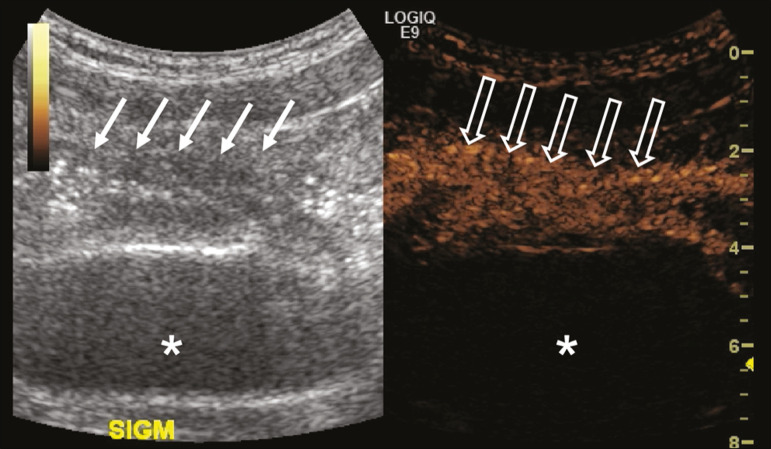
A 15-year-old patient, under follow-up treatment for Crohn’s disease, with abdominal pain and suspected exacerbation of the disease. Images after intravenous injection of microbubble contrast medium, showing wall thickening of the sigmoid loop (arrows) on B-mode ultrasound; in the contrast-specific mode, note the intense microbubble contrast parietal enhancement of the loop (open arrows). The anechoic structure at the bottom of the image is the full bladder (asterisks).
